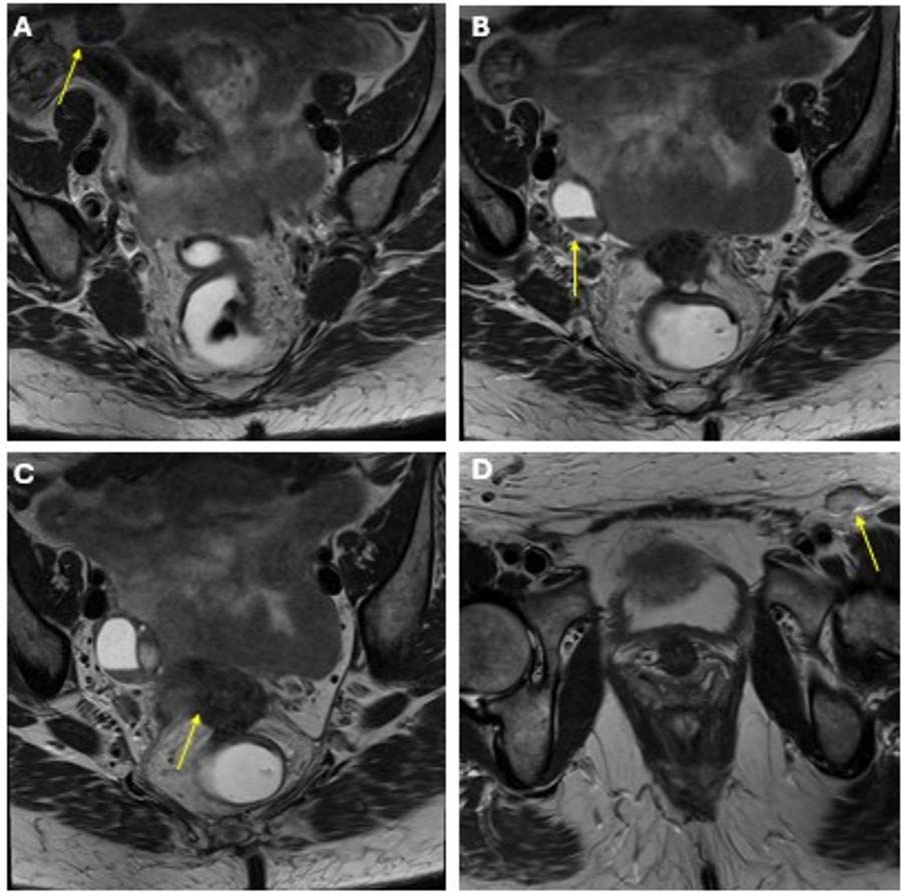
MRI obtained status post 20 cycles of adjuvant pembrolizumab with a stable-appearing mass abutting the right rectus abdominis (A), right adnexal masses (B), a mass along the anterior peritoneal reflection abutting the rectum (C), and a left inguinal lymph node (D).
